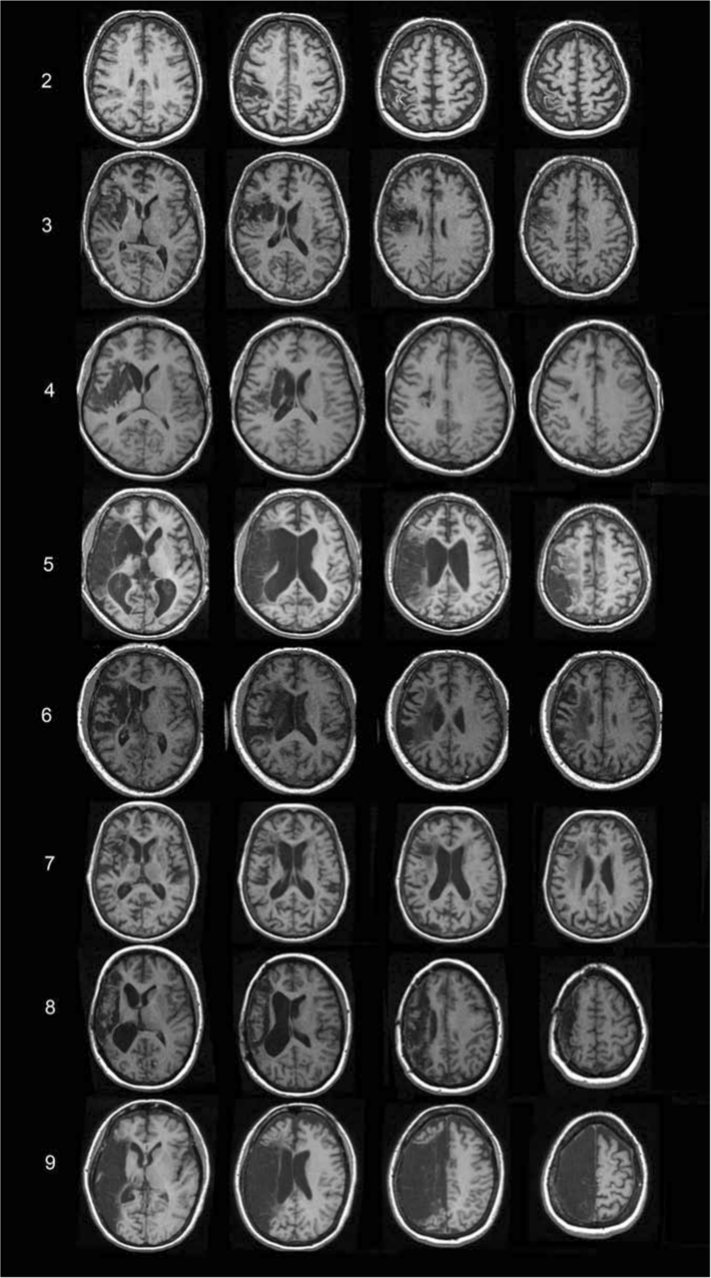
Four representative slices of stroke lesion territory are shown for 8 of 9 stroke patients. The numbers along the left side of Figure 2 correspond to the ID numbers in Tables 1 and 2. Although processed lesion data was available for patient #1, the raw MR images were not locatable.
